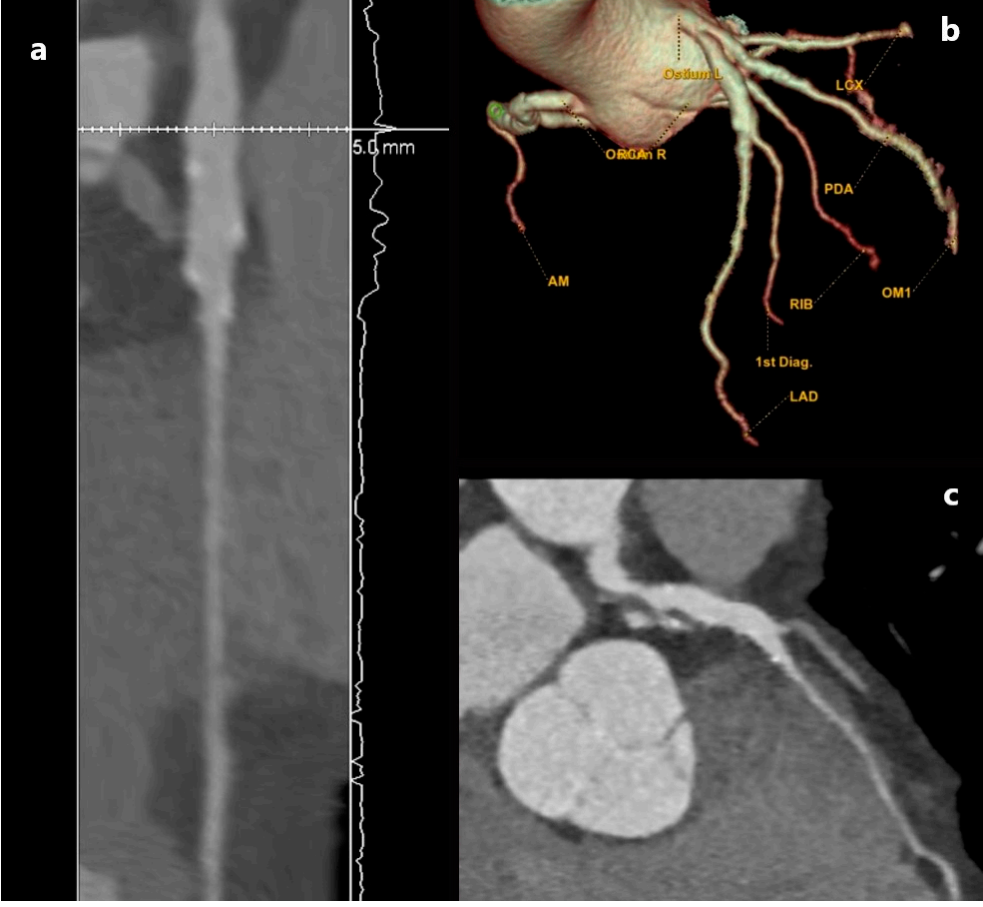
(a) MD CT coronary angiography angiographic (b) three-dimensional-volume rendered image and (c) planar view images, showing a left anterior descending coronary artery fusiform aneurysm. The presented patient underwent MDCT coronary angiography due to chest pain during exertion and shortness of breath. As all presented plaques caused less than 70% lumen reduction on examination, noted symptoms were due to either the presented aneurysms or concomitant myocardial bridges (seen in post-aneurysmal segment in (b)). Conservative treatment and radiological follow-up was indicated.
